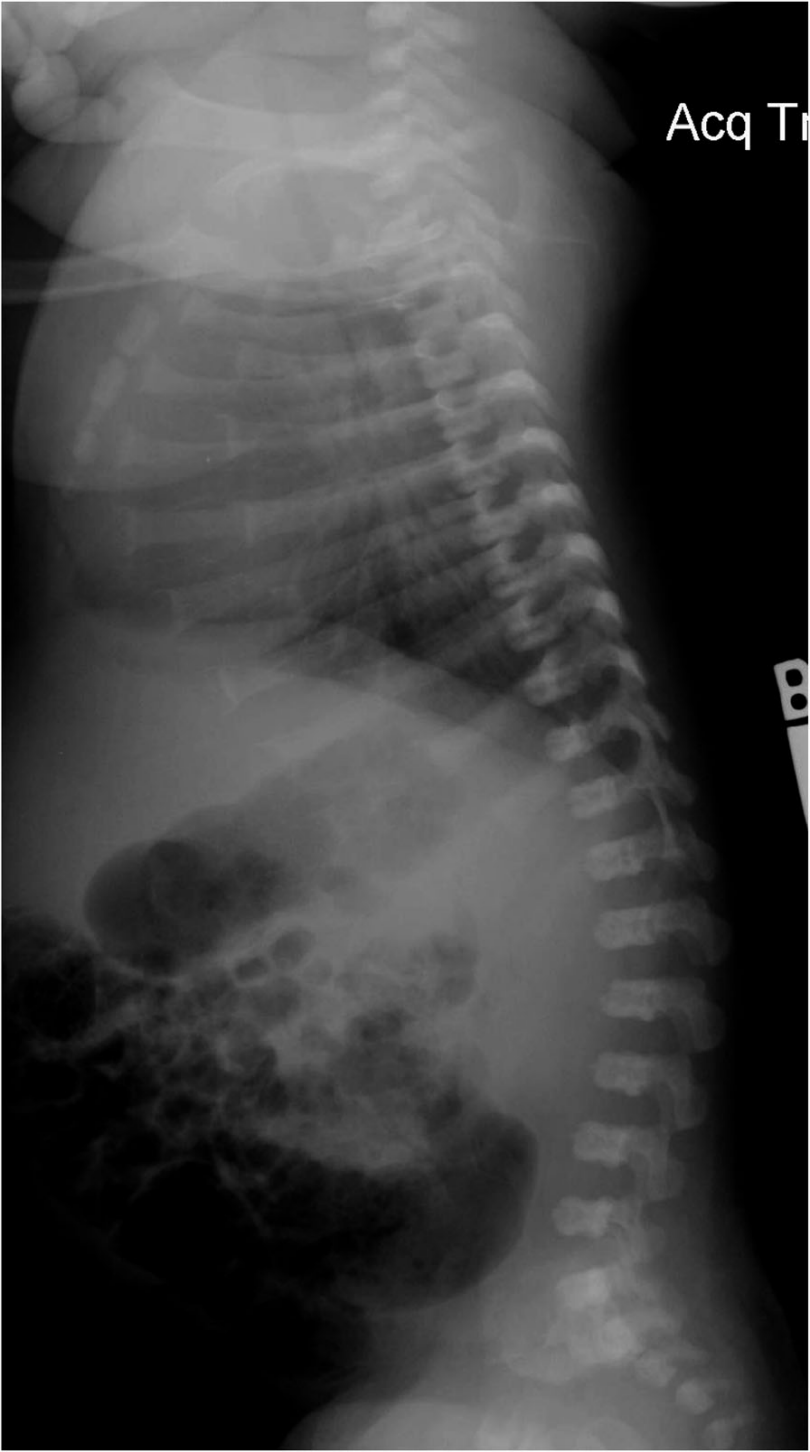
Lateral radiograph of the spine of an infant with achondroplasia. While there is obvious kyphosis, there are no secondary changes of the vertebral bodies at the apex of the curve
